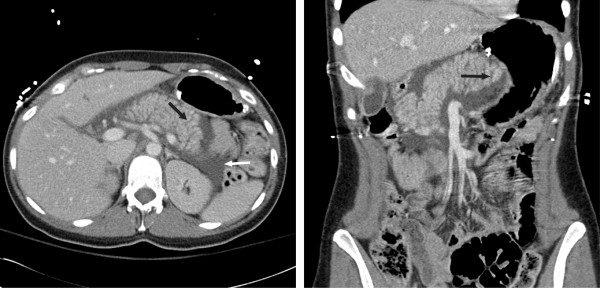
Enhanced axial and coronal computed tomography images through the abdomen demonstrate diffuse enlargement of the pancreas. Numerous linear hypodensities in the pancreas have the appearance of widened pancreatic folds (black arrows). Simple fluid is localized to the peripancreatic region, within the retroperitoneum (white arrow).
